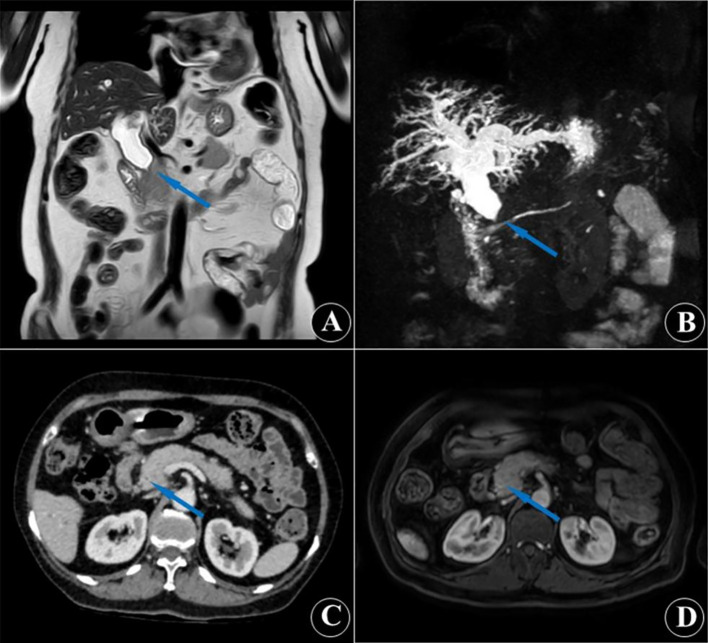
Contrast-enhanced MRI (A, D), MRCP (B) and contrast-enhanced CT (C) showing features of biliary obstruction, including wall thickening and luminal narrowing of the distal common bile duct (blue arrow).
